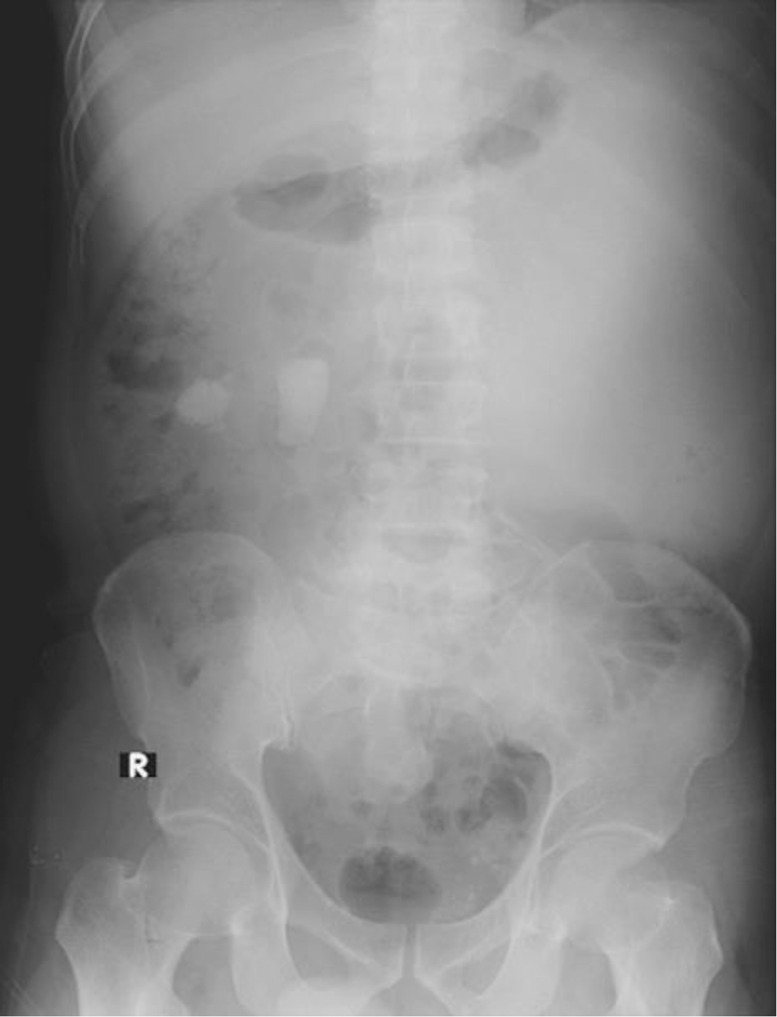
Abdominal X-ray showed ground-glass opacity at the left upper-lower quadrant abdomen accompanied by two oval-shaped opaque shadows at the level of 2nd – 3rd lumbar vertebrae.
